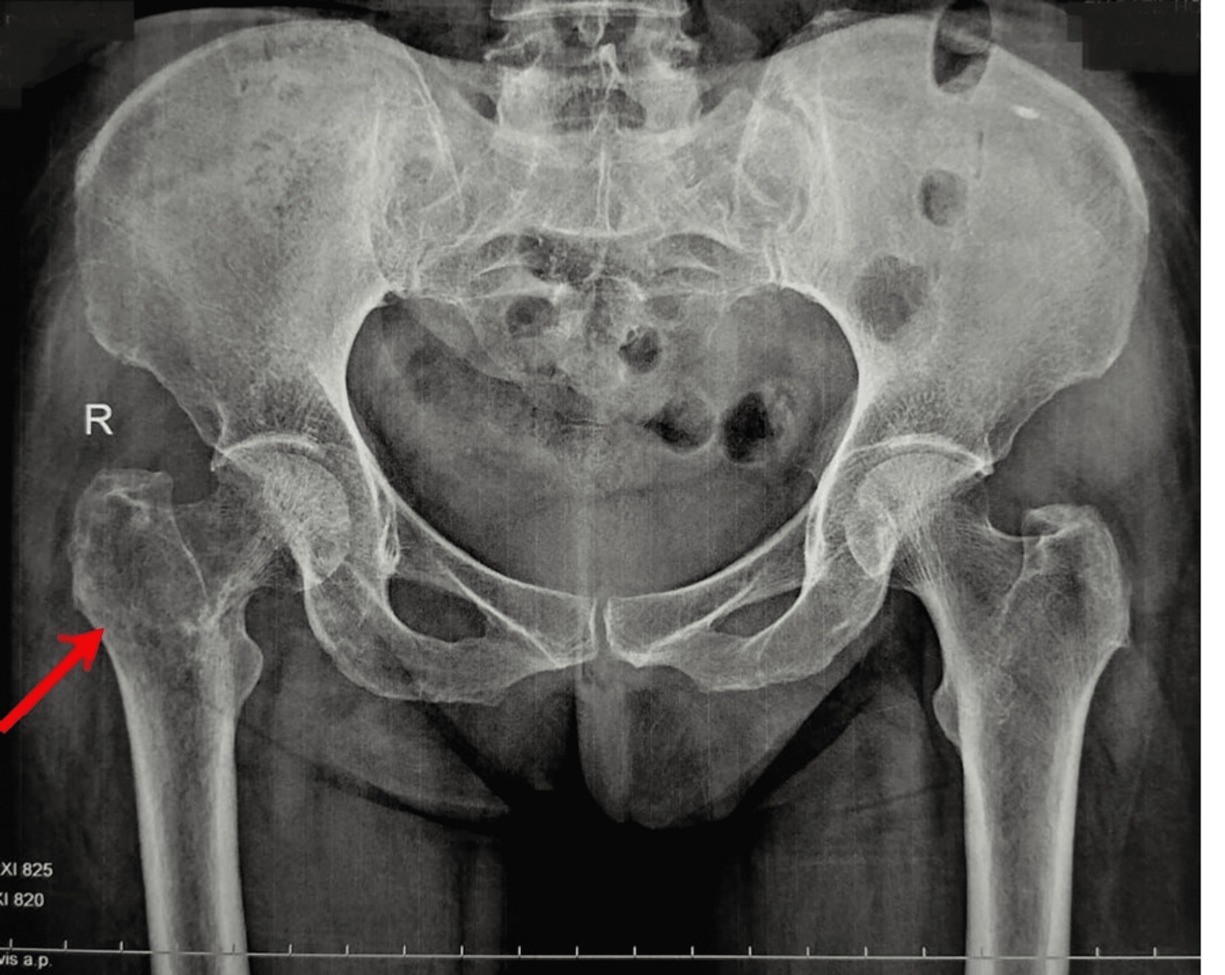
Preoperative X-ray pelvis with both hips showing radioluscent lesion with discontinuity of the cortex of calcar indicating a pathological fracture in the right proximal femur (arrow) The red arrow mark shows a discontinuity in the calcar of the right side indicating a pathological fracture with coxa vara on the right side when compared with the left side of the femur.
